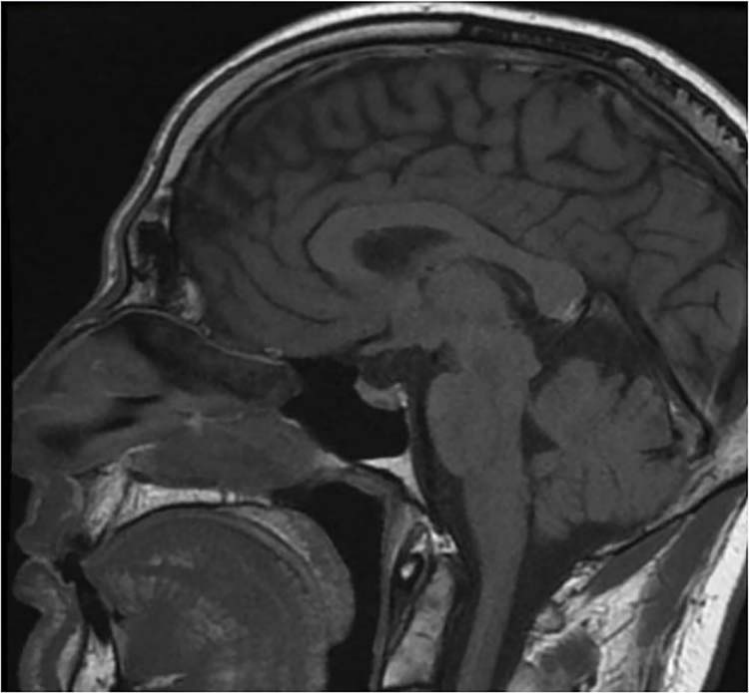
MRI in T1. The detailed MRI study of the diencephalon-pituitary region shows a thinned pituitary gland lying on the sellar floor, with a concave profile. A small expansive, oval, formation with a maximum diameter of 8 mm and height of about 3 mm is located in the median/left paramedian and basal portion of the gland.
